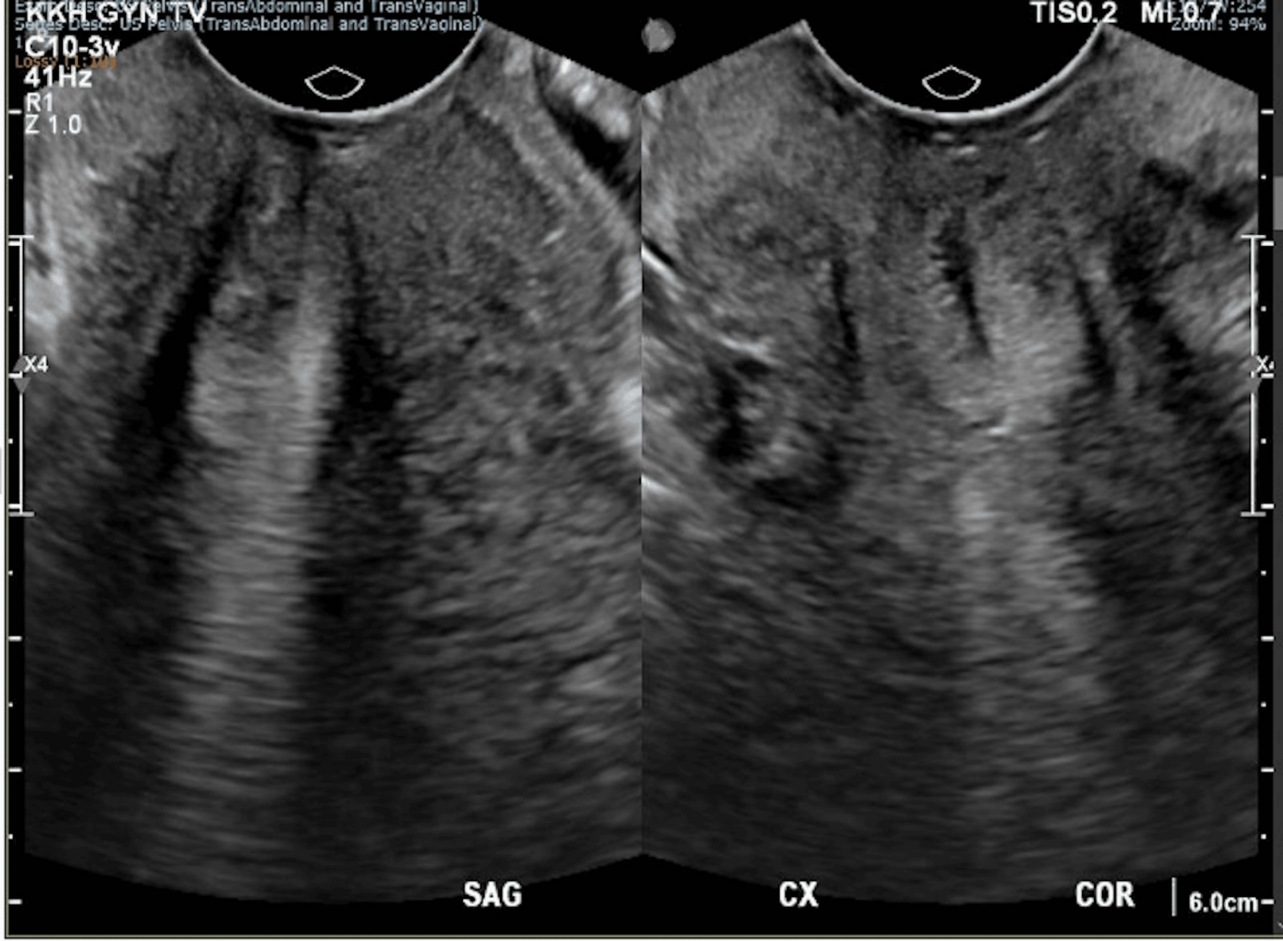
Sagittal (left) and coronal (right) views of posterior cervix on transvaginal ultrasonography post treatment showing resolution of vaginal endometriosis and endometriotic deposits in the Pouch of Douglas.
